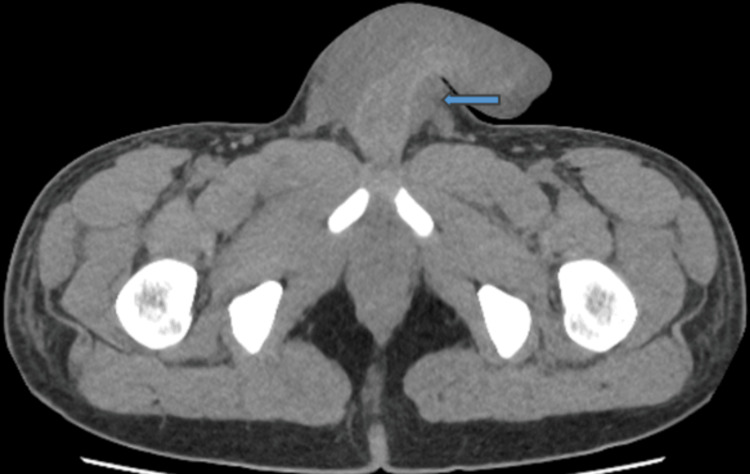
CT scan of the abdomen and pelvis: enhancing peri-urethral fluid collection, indicative of a large peri-urethral abscess.
